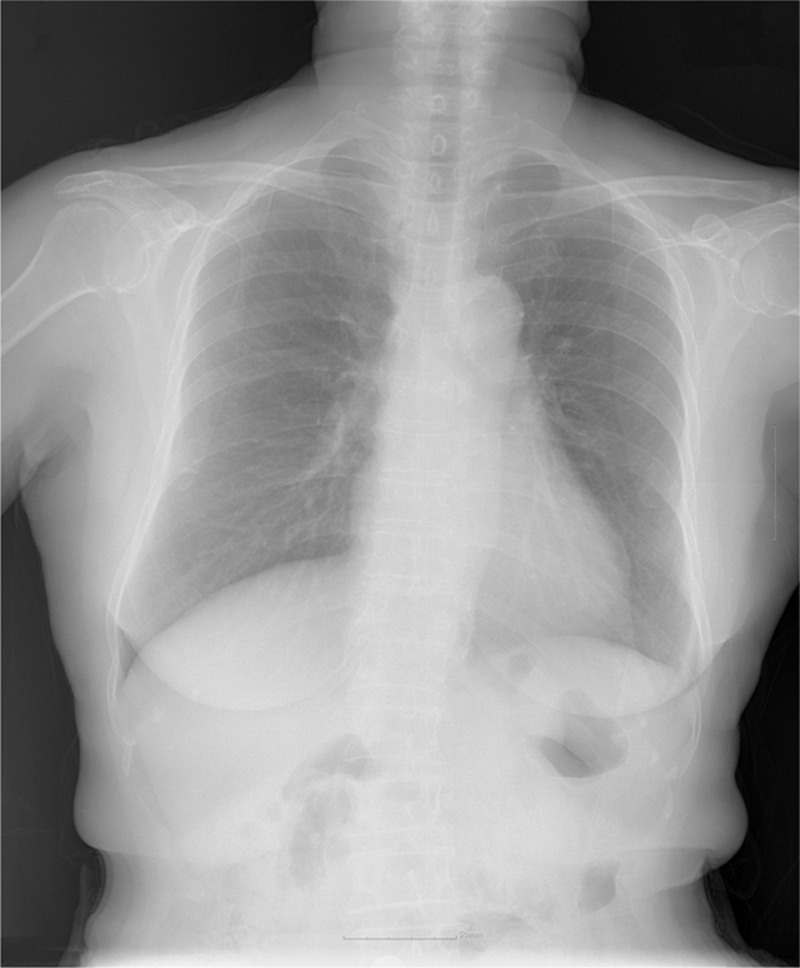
Preoperative chest X-ray.
